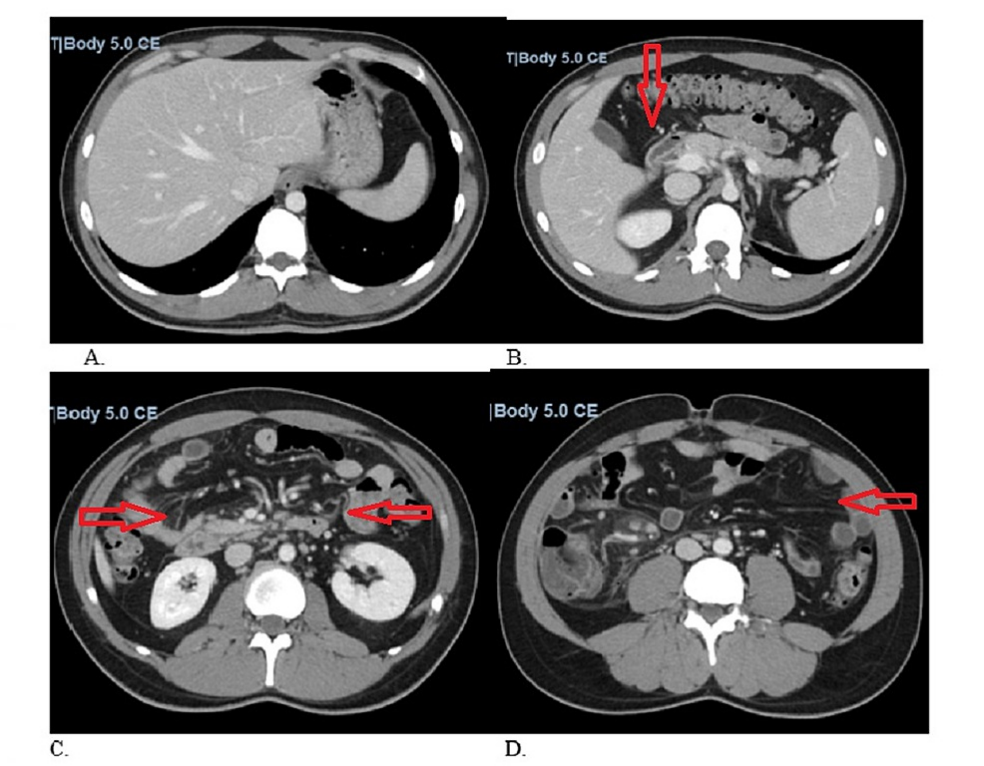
CT of the abdomen and pelvis with intravenous contrast. Axial CT through the abdomen and pelvis with IV contrast. Focal fat along the falciform ligament (A). There is wall thickening of the terminal ileum, cecum, and ascending colon (B). There is mesenteric stranding and lymphadenopathy noted in the right mesentery (C and D). There is no free air, no ascites, and no evidence of gastrointestinal obstruction.
